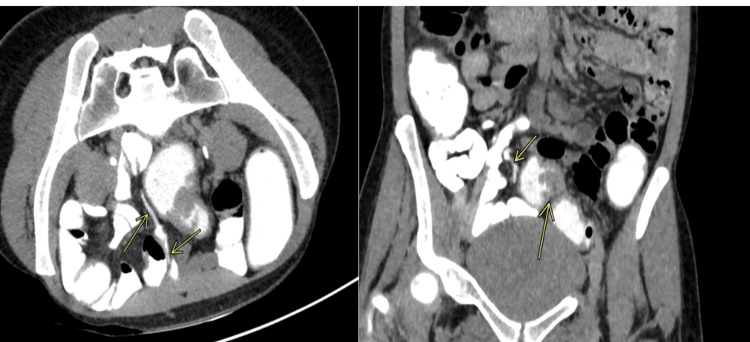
(A, B) Axial and sagittal CT images showing fistula in the abdomen.
